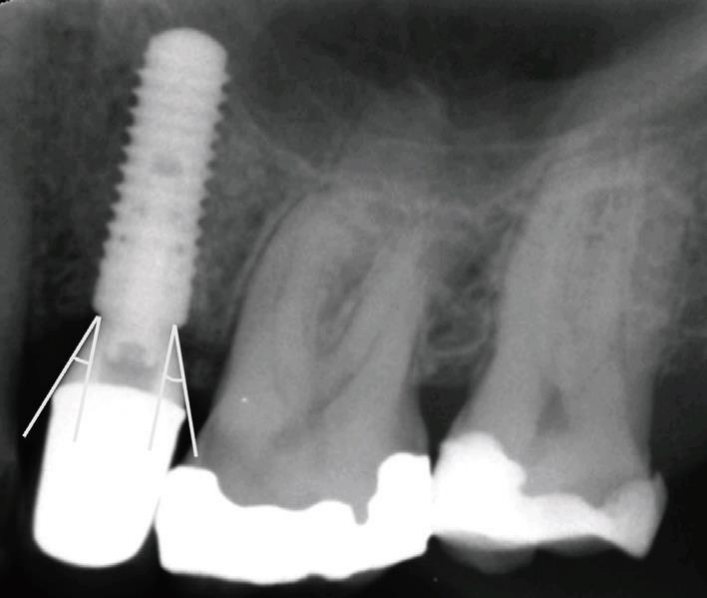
Radiograph showing measurements of the emergence angle relative to the longitudinal axis of the implant.
